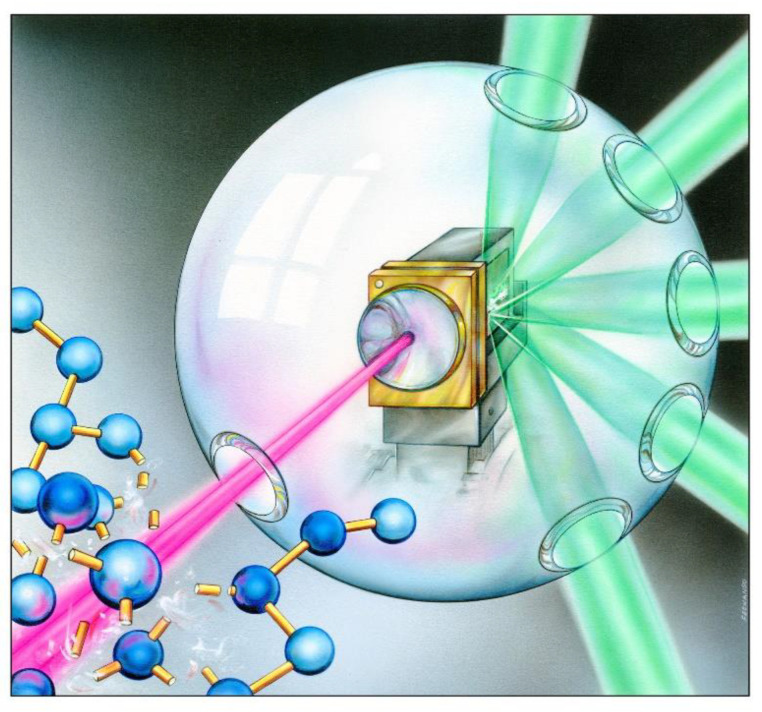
Cover of the January 1987 issue of the famous French Journal *La Recherche* showing an artist’s view on how the laser-produced plasma-based soft-X-ray laser beam (false color: violet) decomposes a molecule [10]. The artist/graphic designer: © Fernando da Cunha (reproduced with artist’s permission).
